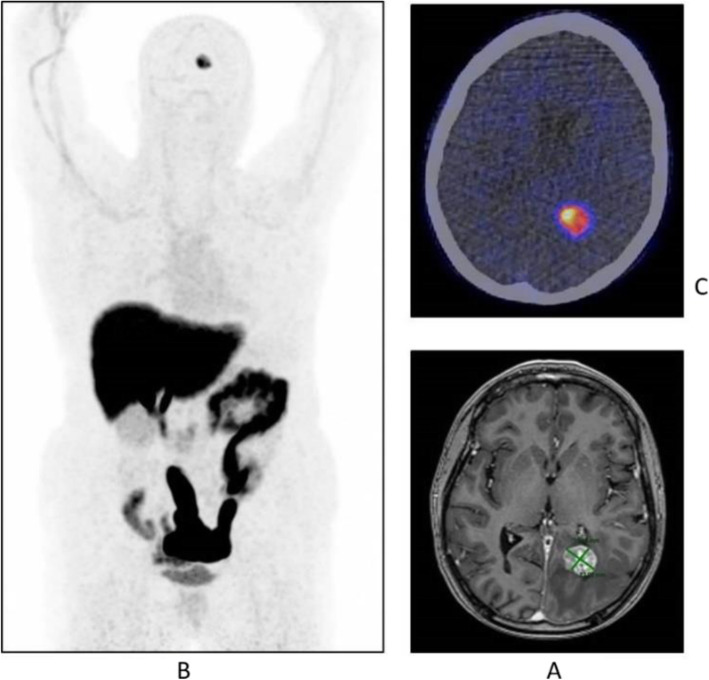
^18^F-fluoroestradiol, breast cancer, characterisation of brain metastasis. *Clinical history*: 53 y.o. woman with history of colon cancer (2004) and breast cancer (2011), ER+, presenting with an 18-mm brain lesion on MRI (a). Biopsy was not possible due to location. *PET/CT findings*: solitary lesion with increased ER expression in the brain in MIP (b), located in the left occipital lobe on fused images (c), suggesting brain metastasis from breast cancer
